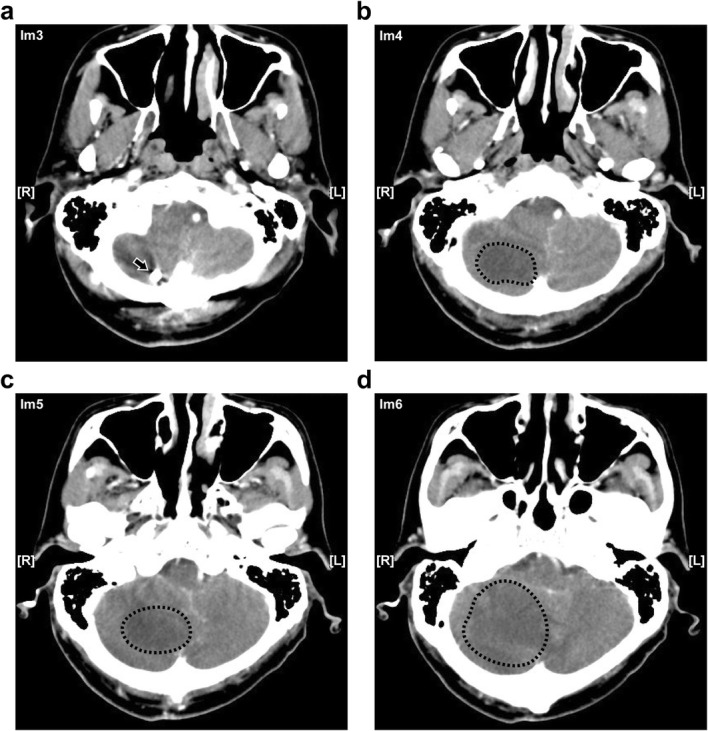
The axial images of four continuous CT of the patient with cerebellar abscess. The four continuous CT images from a to d represented continuous section from the base of the skull to top. The arrow in (a) indicated the position where the screw penetrates the dura mater. Circled area was indicated as brain abscess
